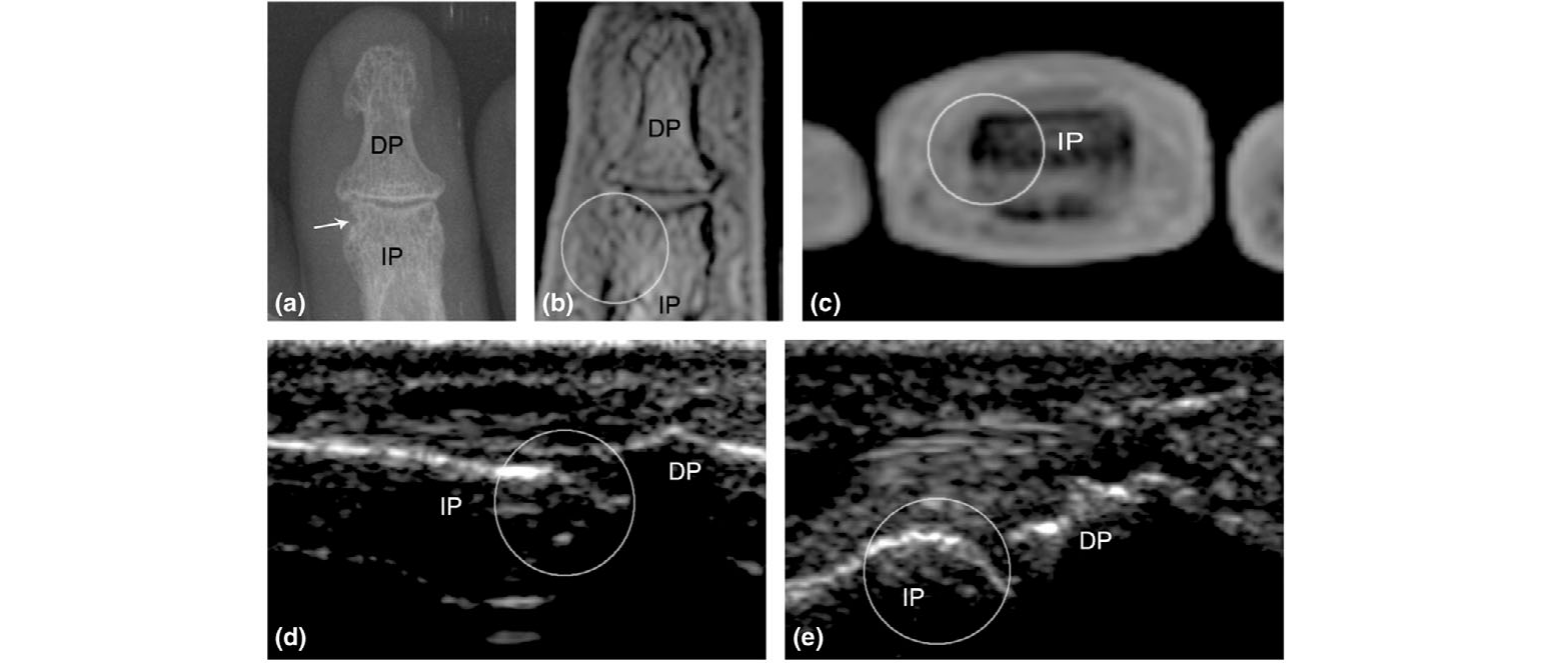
Projection radiography (x-ray) (a) and T1-weighted coronal (b) and axial (c) magnetic resonance imaging (MRI) and ultrasonography (US) in longitudinal dorsal (d) and palmar (e) views of the 3rd distal interphalangeal joint of a patient with psoriasis-associated arthritis. An erosion was scored on x-ray (a) but not on either MRI (b,c) or US (d,e), even though small irregularities were seen. DP, distal phalanx; IP, intermediate phalanx.
